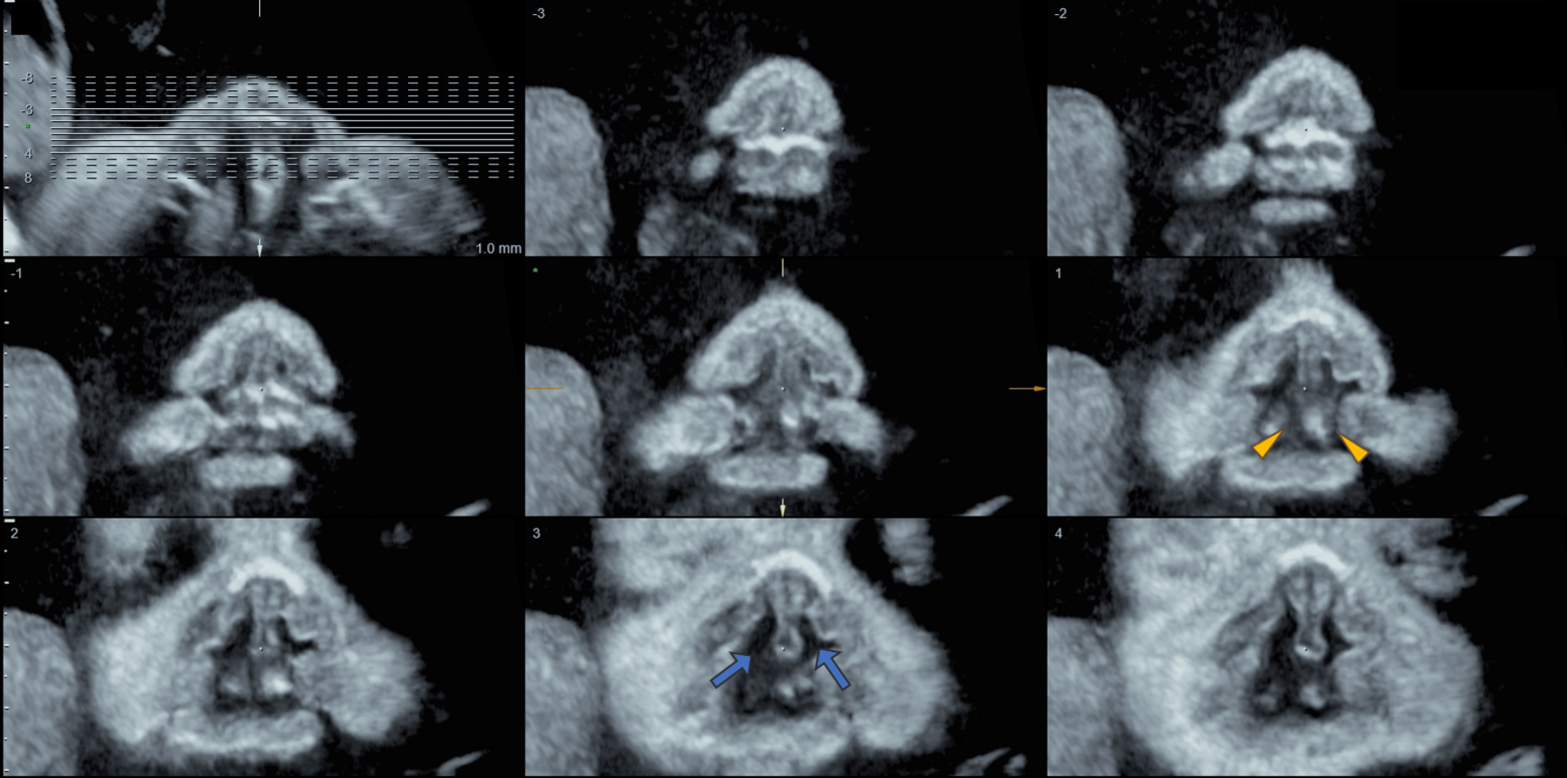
Detection of the cleft jaw using tomographic ultrasound imaging. Tomographic ultrasound imaging was used to scan the coronal section at 1 mm intervals centered on the palatal area, and a cleft jaw was suspected due to the presence of nasal-to-oral cavity traffic (blue arrows) as well as a defect in the alveolar ridge (yellow arrowheads).
